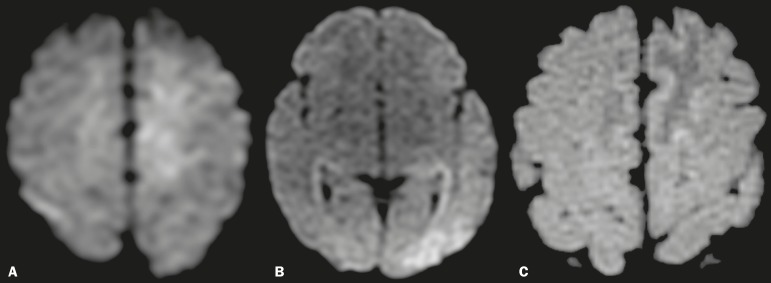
Diffusion-weighted MRI scans of the brain of a newborn, born to a woman
